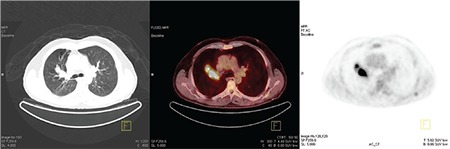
Increased ^18^F-FDG uptake is detected in the lobulated contoured mass lesion at the right upper lung lobe anterior segment.
